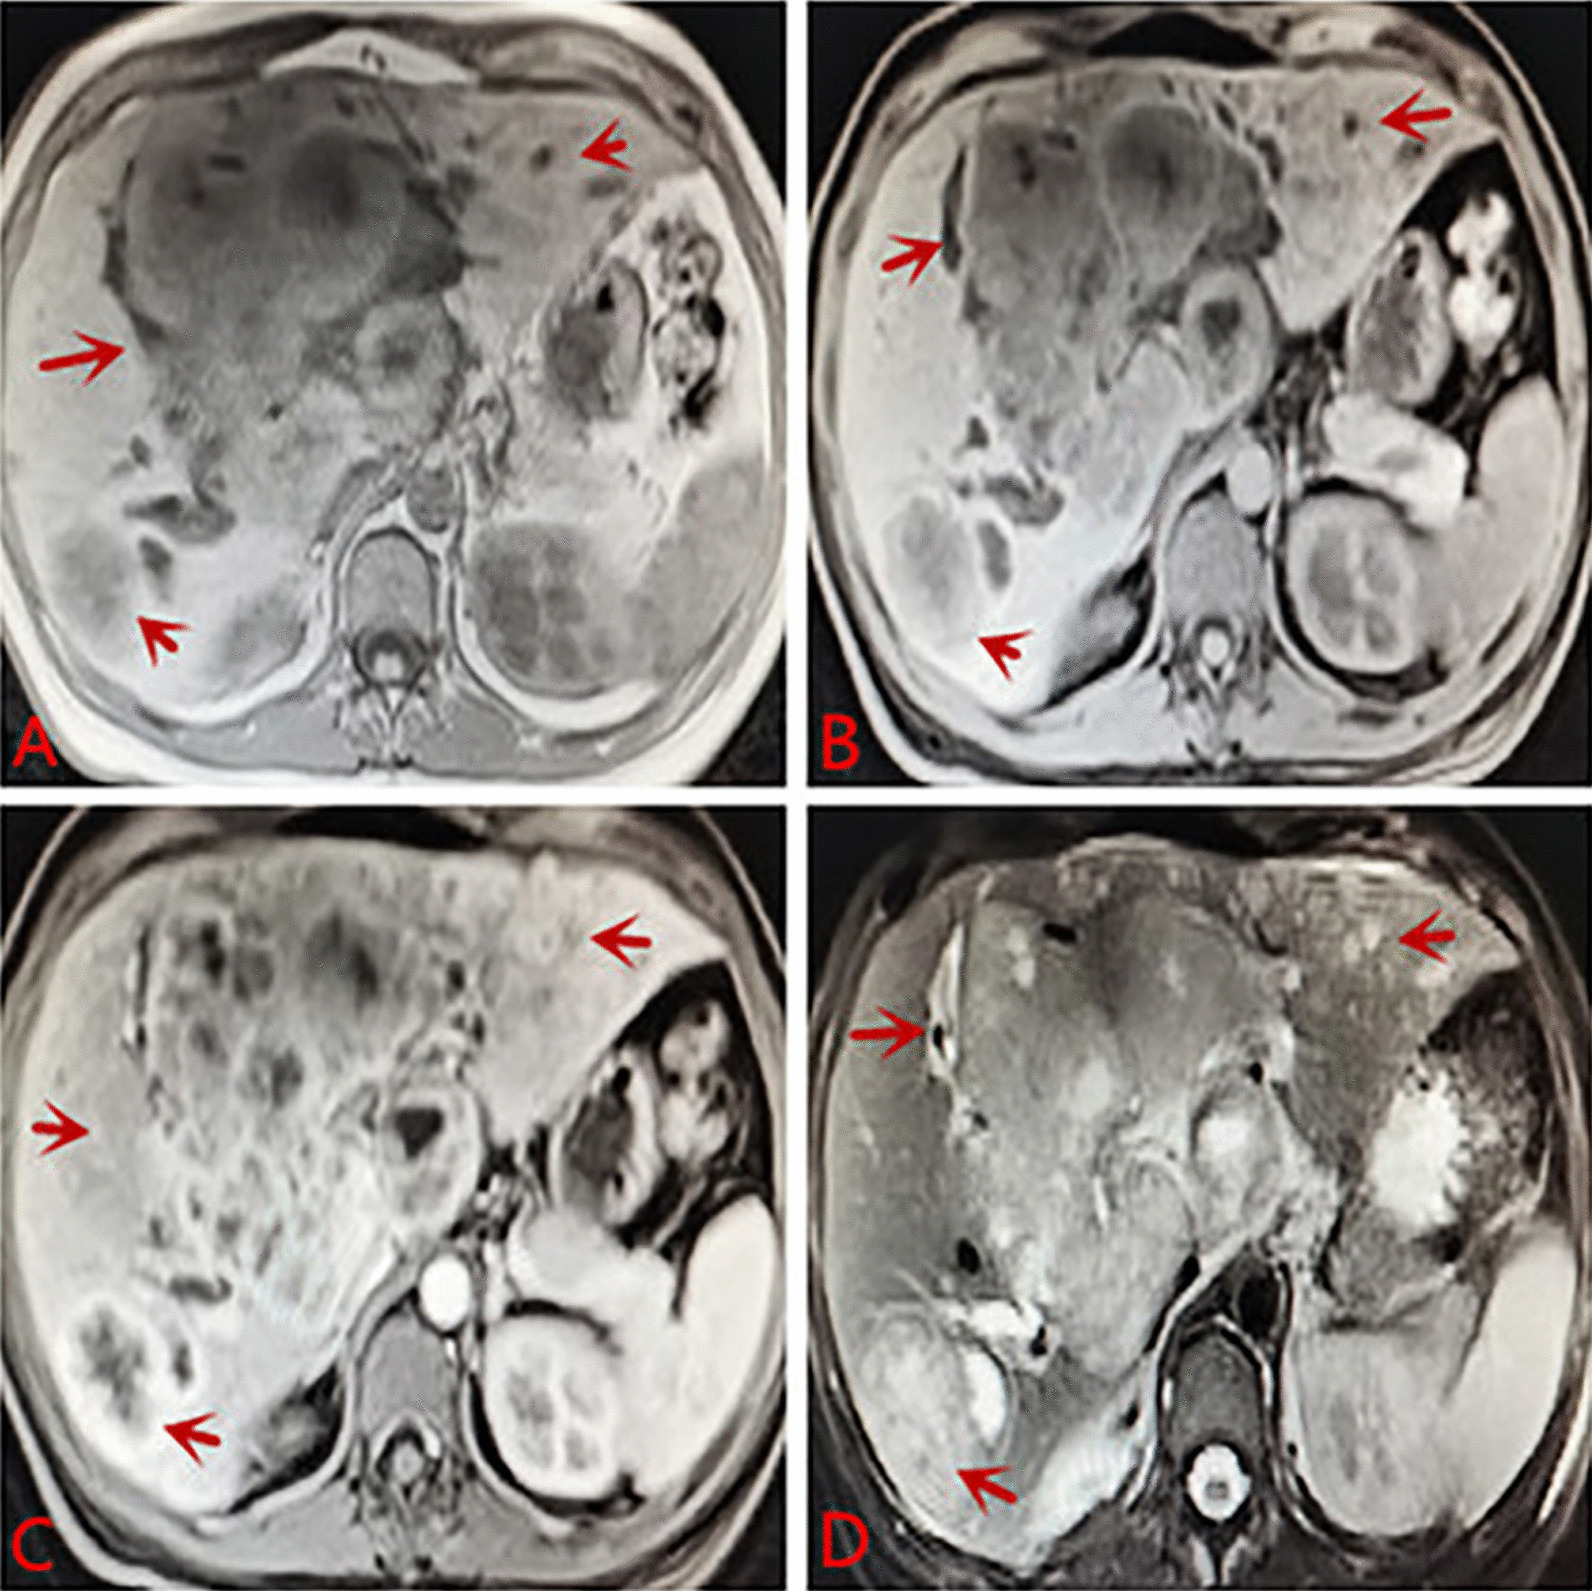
A Plain MRI scan: increased liver volume with multiple nodules and mass shadows inside, showing slightly long T1 and slightly long T2 signals, liquefaction necrosis changes were observed in the center of the lesion, and some lesions appeared to be fused. B–D Contrast-enhanced scans showed mild enhancement of nodular mass shadows and ring-like enhancement changes. Consider malignant tumor combine with intrahepatic multiple metastases. The left branch of the portal vein was not clearly displayed, consider possible involvement. The right branch of the portal vein was suspect been locally invaded, the hepatic vein and the bile duct in the hilar area were not clearly displayed, the bile duct at the upper level was slightly dilated, the left upper quadrant was turbid, consider the possibility of local nodules
